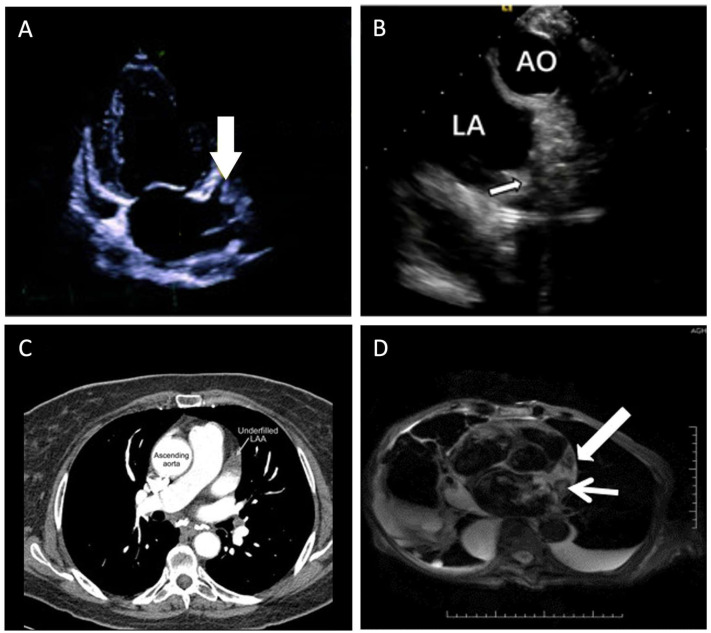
Imaging modalities to evaluate the left atrial appendage. (A) Transesophageal echocardiography—apical two chamber view during transthoracic echocardiogram without contrast showing thrombus in LAA [25]. (B) Intracardiac echocardiography—view from right ventricular outflow tract [26]. (C) Multi-detector computed tomography—axial image using MDCT showing underfilled or “abnormal” LAA with failure of contrast to fill the LAA (arrow) [27]. (D) Cardiac magnetic resonance—CMR paraxial view demonstrating T2-weighted image of the LA and LAA with fresh clot (narrow arrow) showing higher signal intensity than the old clot with reduced central signal intensity (broad arrow) [28]. *AO—aorta, LA—left atrium, LAA—left atrial appendage, MDCT—multi-detector computed tomography, CMR—cardiac magnetic resonance*.
